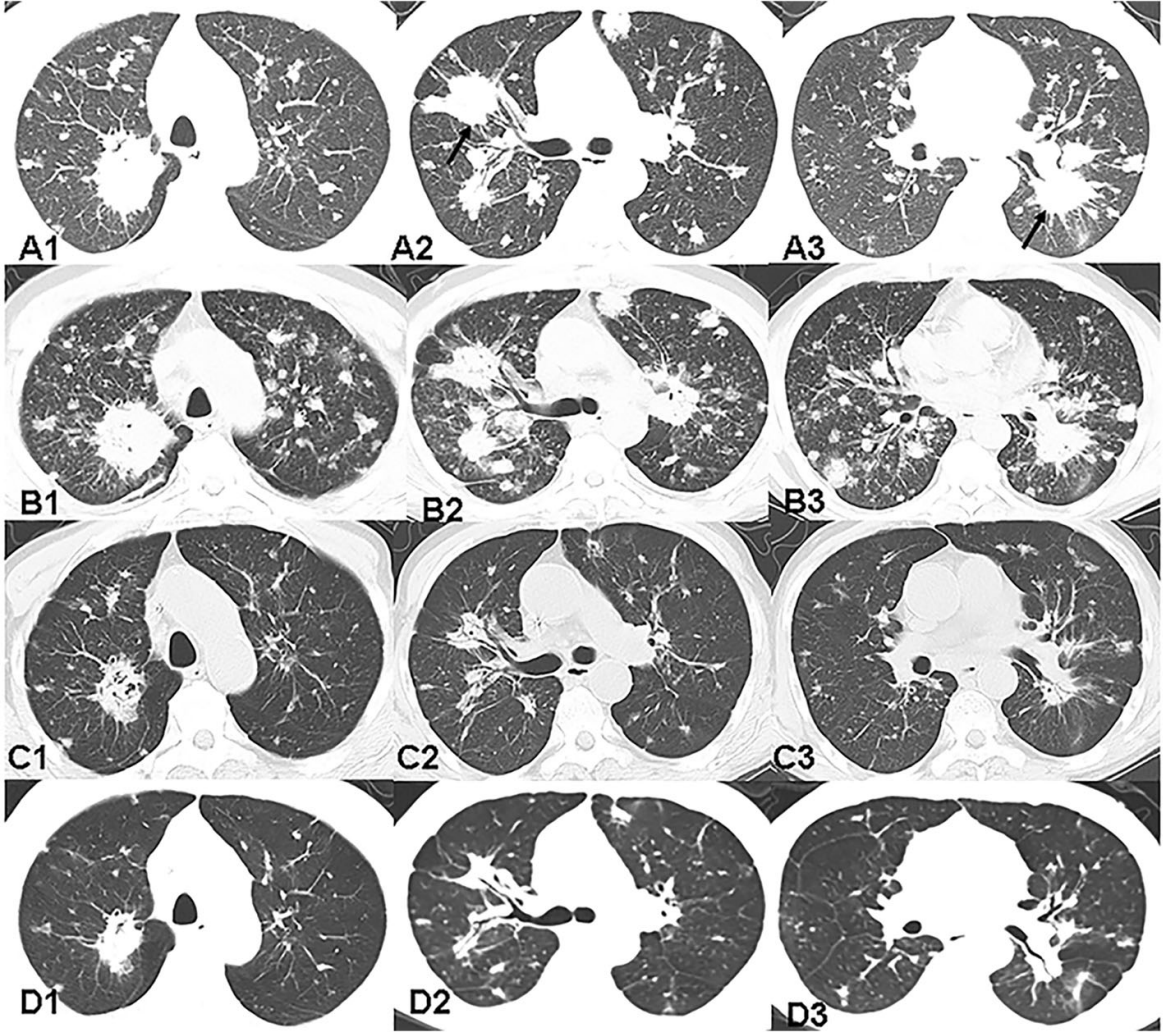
A1-A3 was CT images of lung oftheThoracic Hospital on October 25, 2017, showing multiple spherical lesions in both lungs. Some lesions were closely related to the bronchus (black arrows), and most of the other lesions were randomly distributed. B1-B3 showed the number of lung nodules increased before antifungal treatment. C1-C3 were re-examination after 2 weeks of treatment, showing lesions in the lung significantly reduced. D1-D3 were images follow up 3 months of oral fluconazole 400 mg/d, showing that the lung lesions were further reduced
